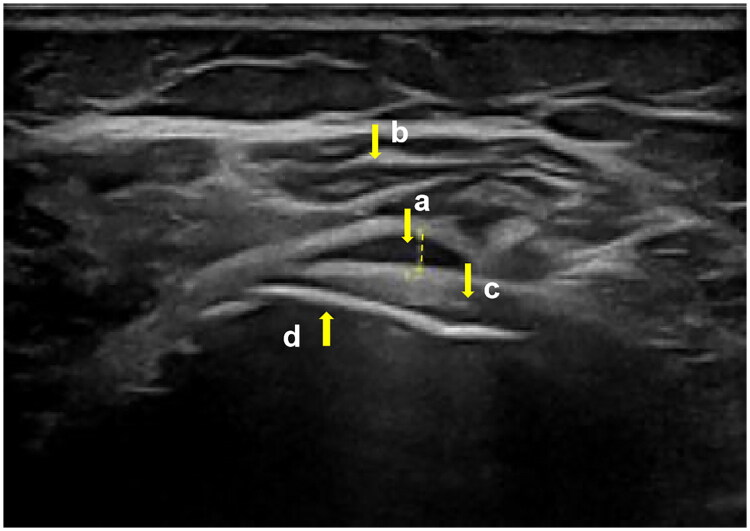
An ultrasound image of SAB. (a) Thickened and distended subacromial bursa containing anechoic fluid; (b) Deltoid muscle; (c) Humeral head; (d) Acromion.
